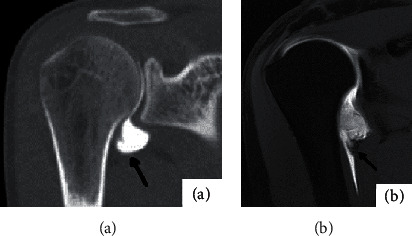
Male, 24 years old, with recurrent anterior dislocation of right shoulder for 1 year. (a) shows the coronal bone window image of CTA in the right shoulder. The shape of the axillary capsule is irregular in arrow. (b) shows the right shoulder MRA coronal T1WI-FS image. The arrow shows the inferior glenohumeral ligament with nonunion of the fibers on the humerus side, retraction of the broken end, and partial contrast agent leakage.
